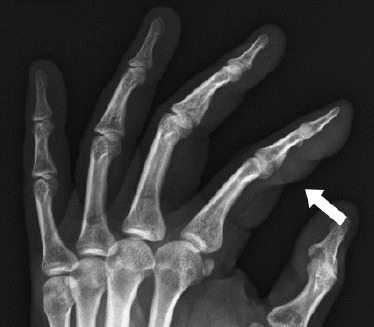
Plain left hand radiograph showing a soft tissue shadow over the index finger with no bony involvement
